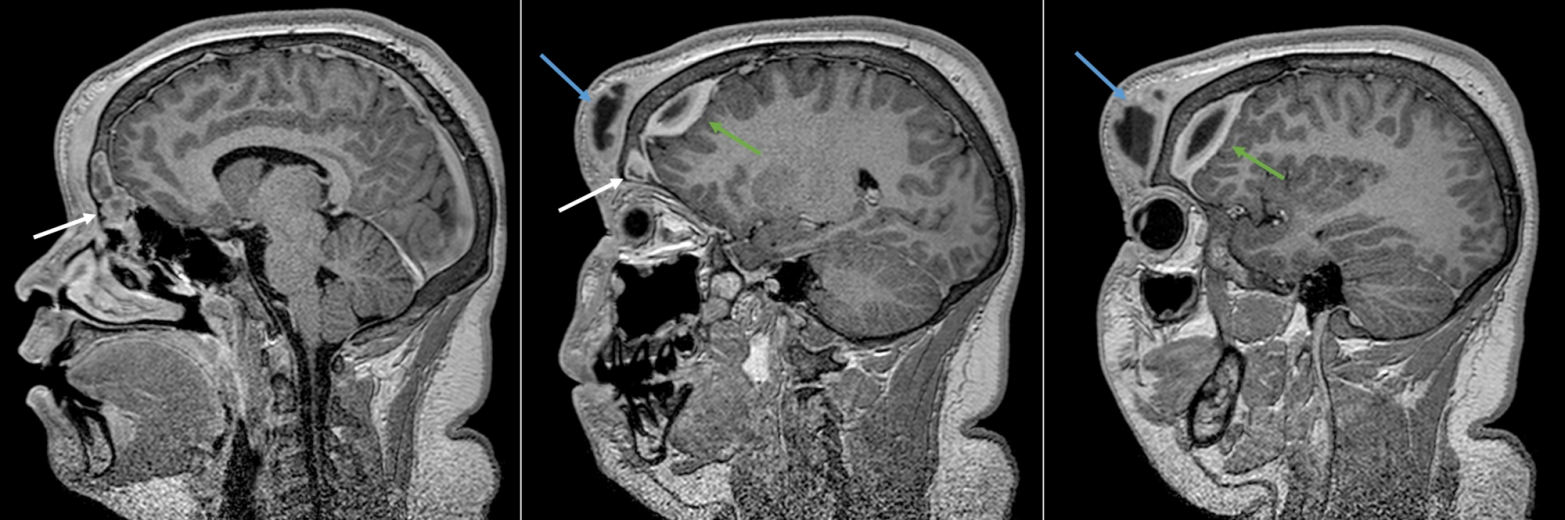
Preoperative MRI of the head: parasagittal reconstruction Parasagittal slices of the preoperative gadolinium-enhanced magnetic resonance imaging (MRI) of the head. In the left and middle images, there is an enhancing lesion filling the left frontal sinus (white arrow) and extending both epidurally (middle and right images - green arrow) and to the periosteum (blue arrow).
